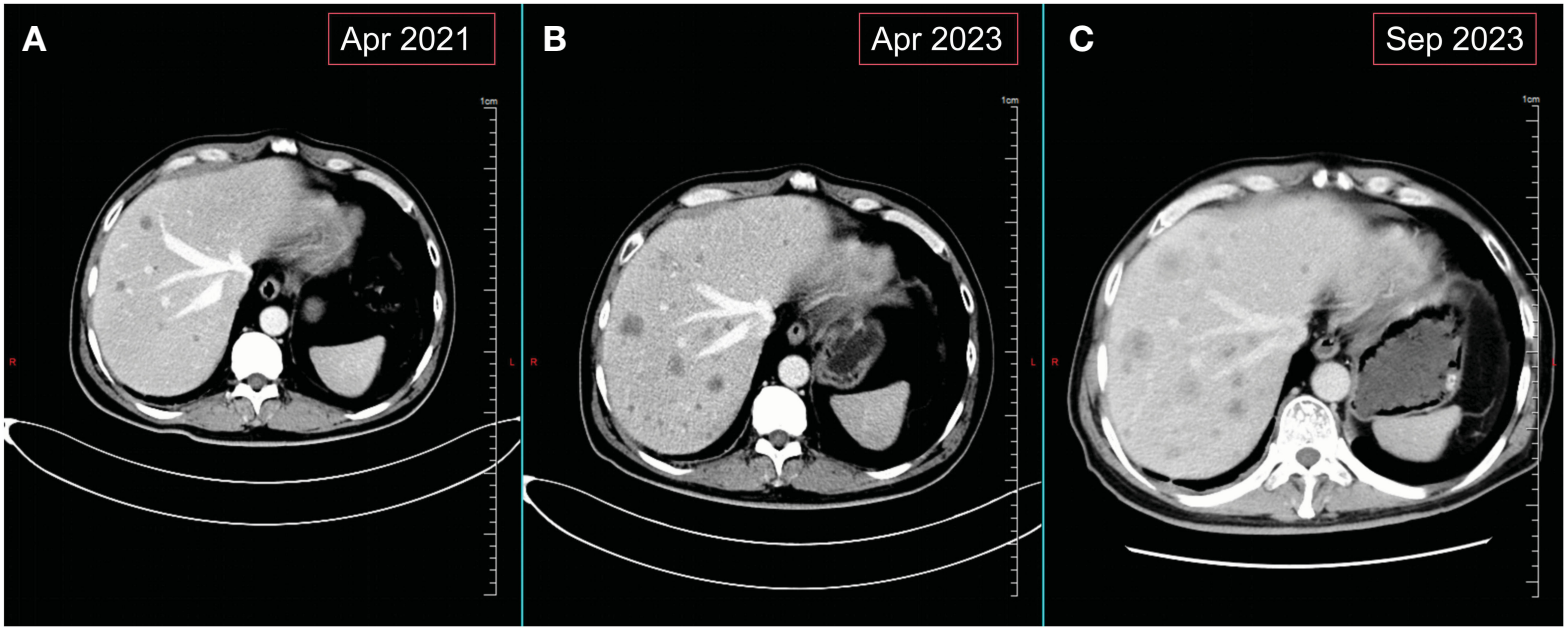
Changes in liver metastases on abdominal CT. (A) Images of liver metastases before monotherapy with crizotinib. (B) Images of the liver at the time of disease progression following treatment with TGRX-326. (C) Hepatic imaging of disease progression following use of 4 courses of chemotherapy combined with immunotherapy for SCLC.
